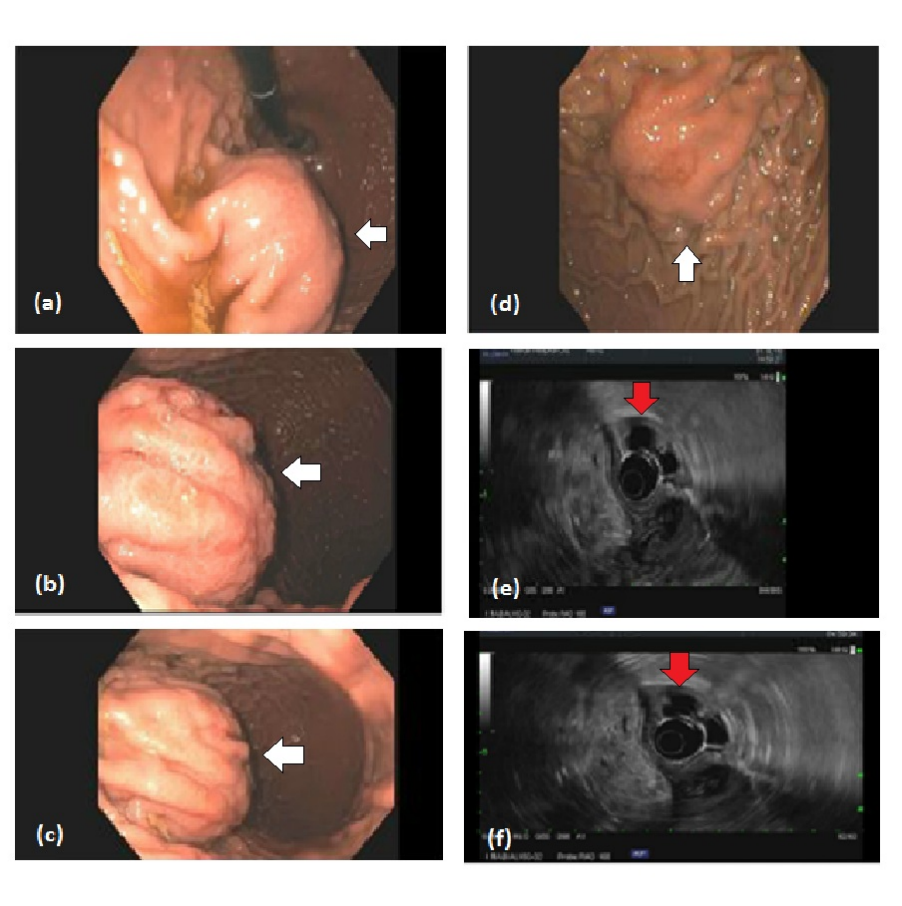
Esophagogastroduodenoscopy (EGD)/endoscopic ultrasound (EUS) of pancreatic pseudocyst. (a-d) EGD demonstrating submucosal bulge from extrinsic compression on the stomach in the proximal gastric body and fundus, there is also small clean-based ulcer on the top of this bulge (white arrows). (e-f) EUS showing anechoic lesion with hyperechoic shadowing suggestive of pseudocyst with bleeding that was identified in the pancreatic tail (red arrows).
